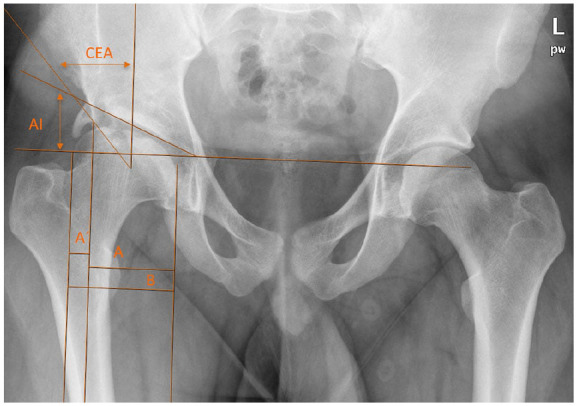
Postoperative radiograph and radiographic measurements 10 years after Chiari pelvic osteotomy for severe LCPD of the right hip. AI, acetabular index; CEA, centre-edge angle; FHC, femoral head coverage percentage = A/B × 100; RI, Reimer’s index = A’/B × 100.
